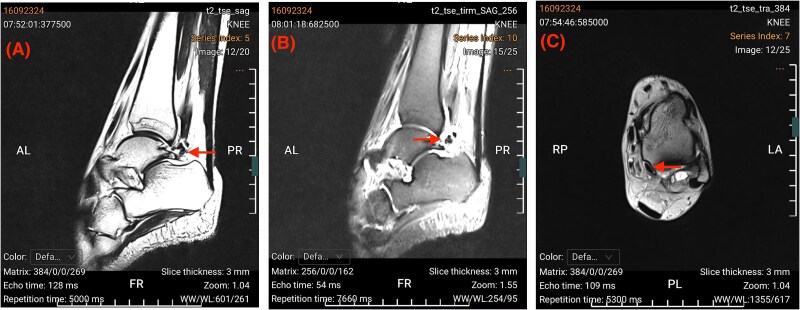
Preoperative MRI of the right ankle. (A) Sagittal T2-weighted image demonstrating a fragmented os trigonum posterior to the talus (arrow). (B) Sagittal fat-suppressed Turbo Inversion Recovery Magnitude image showing bone marrow oedema within the os trigonum fragments and surrounding soft-tissue inflammatory change (arrow). (C) Axial T2-weighted image demonstrating fluid surrounding the FHL tendon sheath (arrow).
